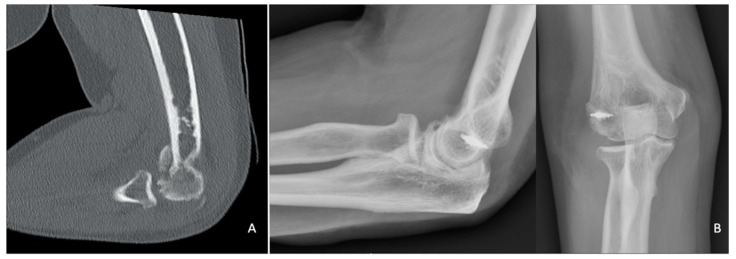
(A) Preoperative CT scan and (B) post-operative X-rays of a Bryan–Morrey Type II fracture treated with fragment excision and lateral collateral ligament repair using a suture anchor.
